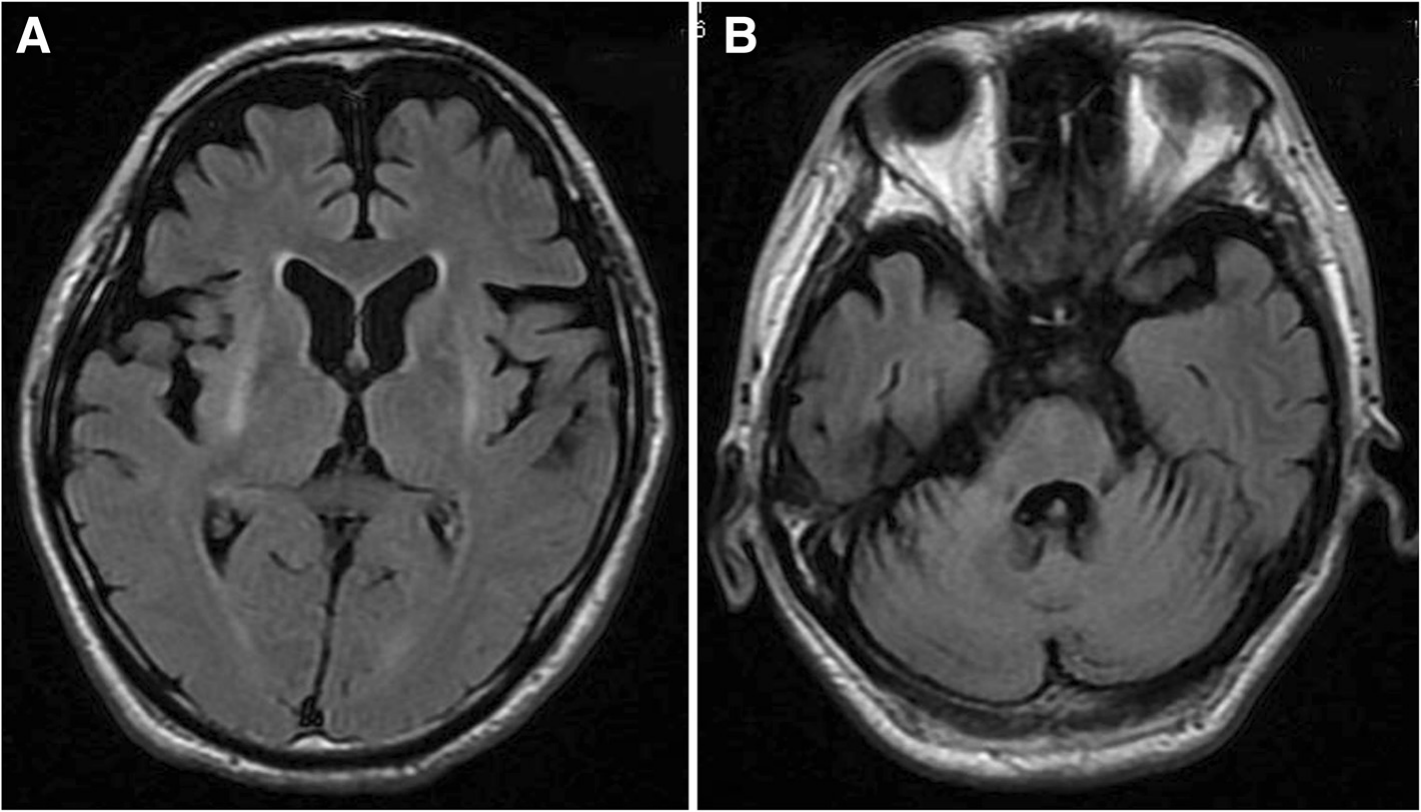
Fluid attenuated inversion recovery images on brain MRI. A, B. Fluid attenuated inversion recovery images on MRI demonstrated linear high signal intensity of putamen bilaterally and mild cerebellar and pontine atrophy.
